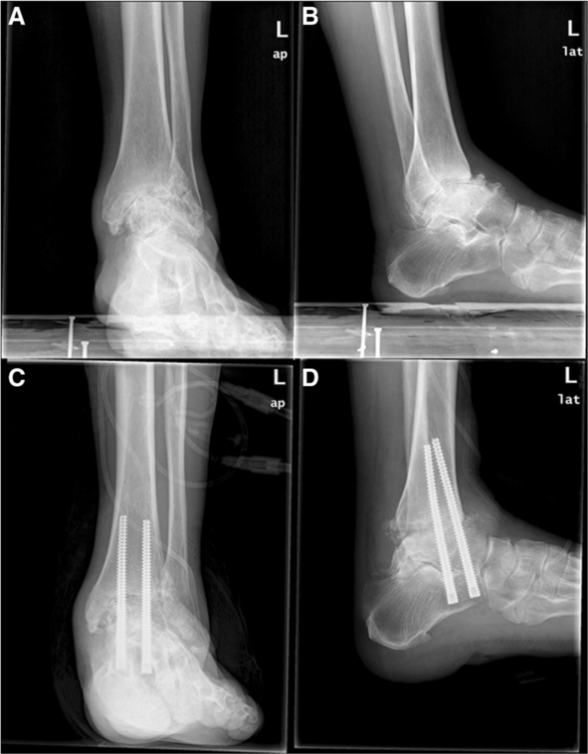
Preoperative (a, b) and postoperative (c, d) radiographs after tibiotalocalcaneal arthrodesis with headless compression screws
